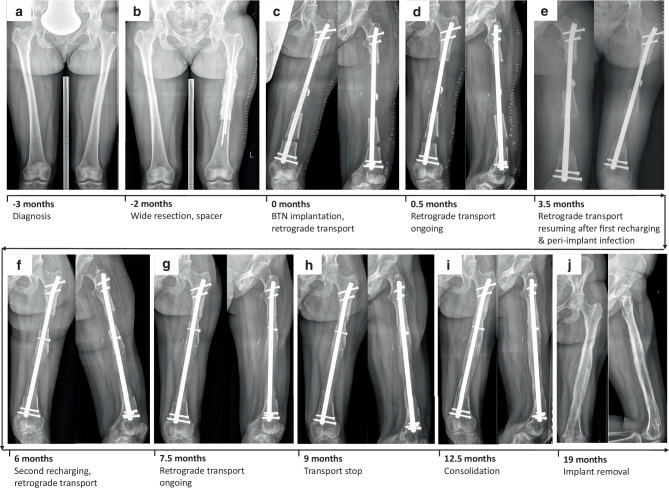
Clinical course of patient 2 (30-year-old female patient with low-grade periosteal osteosarcoma of left femoral diaphysis. X-ray images of both femurs of patient 2 in anterior-posterior (a.p.) plane taken preoperatively (a) and after resection and spacer implantation (b). X-ray images in a.p. and lateral planes directly following bone transport nail (BTN) implantation (c), at 0.5 months (d) 3.5 months (e), 6 months (f) and 7.5 months (g) with bone transport ongoing, at 9 months when bone transport stopped (h), at 12.5 months when bony consolidation had taken place (i), and at latest follow-up 19 months after BTN implantation, when implant removal had taken place and complete bony consolidation was visible (j)
